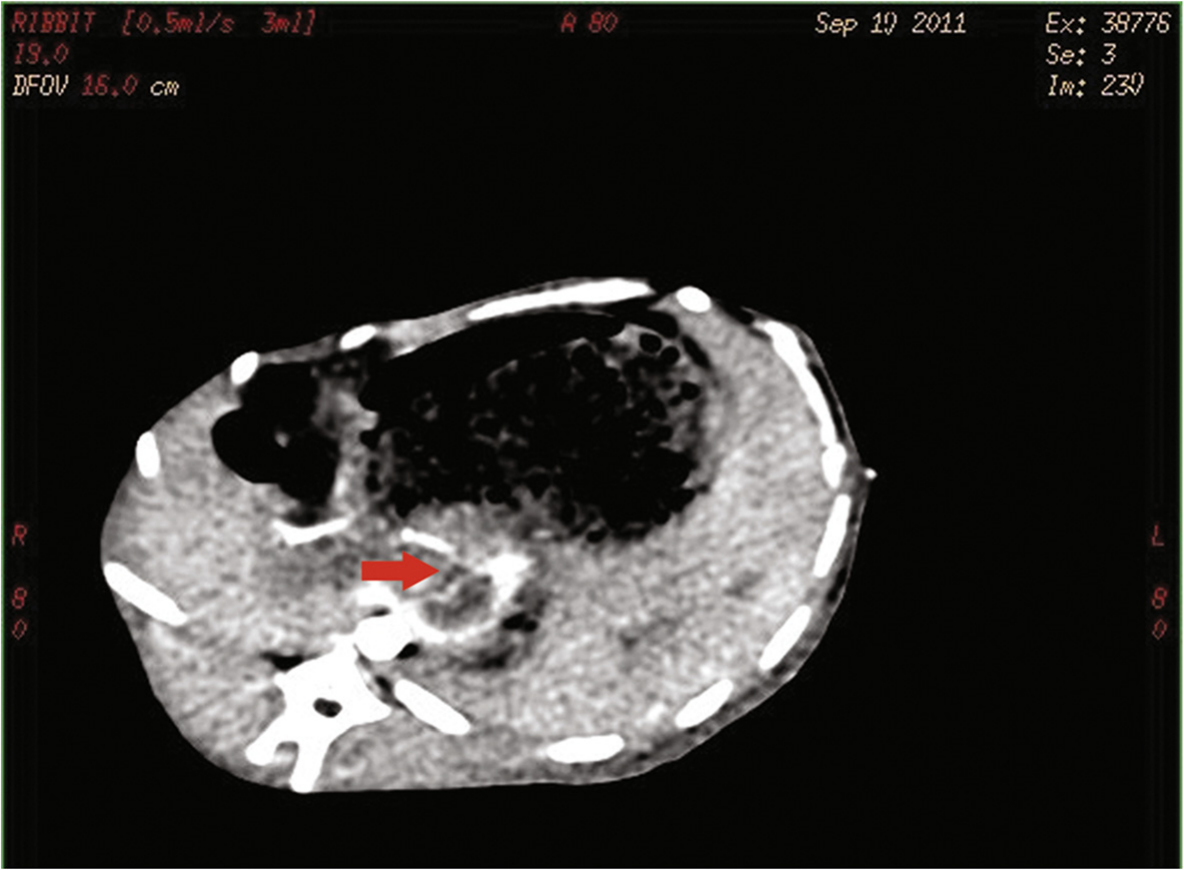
Venous phase contrast enhanced CT scan of PVTT. Note: the red arrow showed expansion of the portal vein, in which filling defect and mild enhancement of xenograft tumor could be observed, indicating successful tumor implantation. CT: Computed Tomography.
